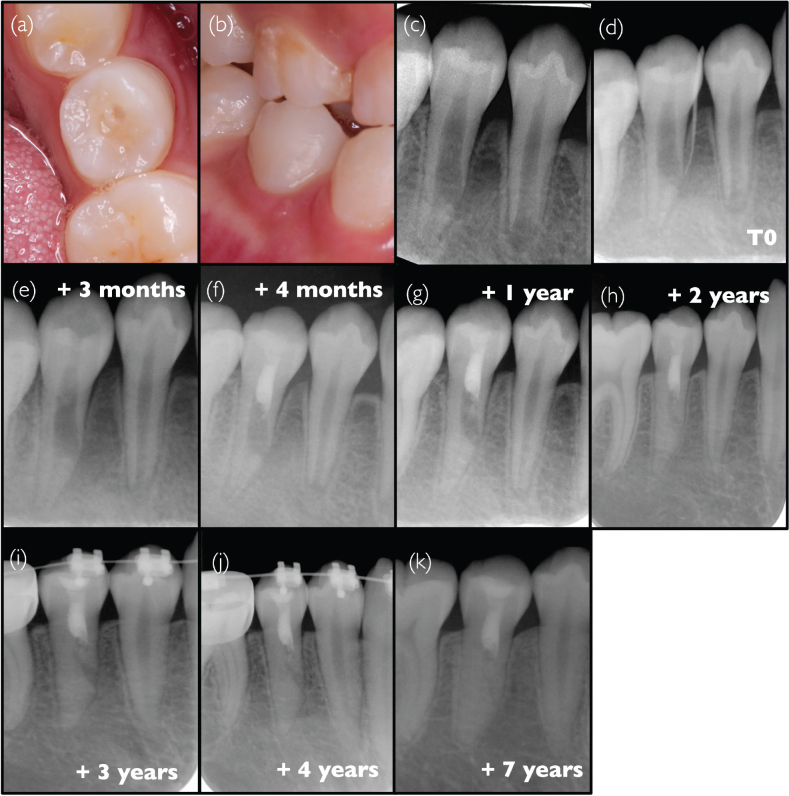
Clinical case #1 (A and B) Pre-operative intraoral photographs and (C) pre-operative radiograph. (D) pre-operative radiograph showing a gutta-percha point inserted into the sinus tract. (E) Radiograph demonstrating the situation after the application of a calcium hydroxide medication. (F) Radiograph following the completion of the revitalisation procedure. (G–K) Follow-up radiographs taken at 1 year and up to 7 years after the initial visit.
